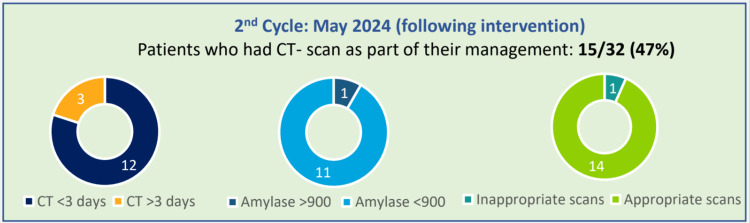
Results of the second cycle of the audit after intervention CT: computed tomography
